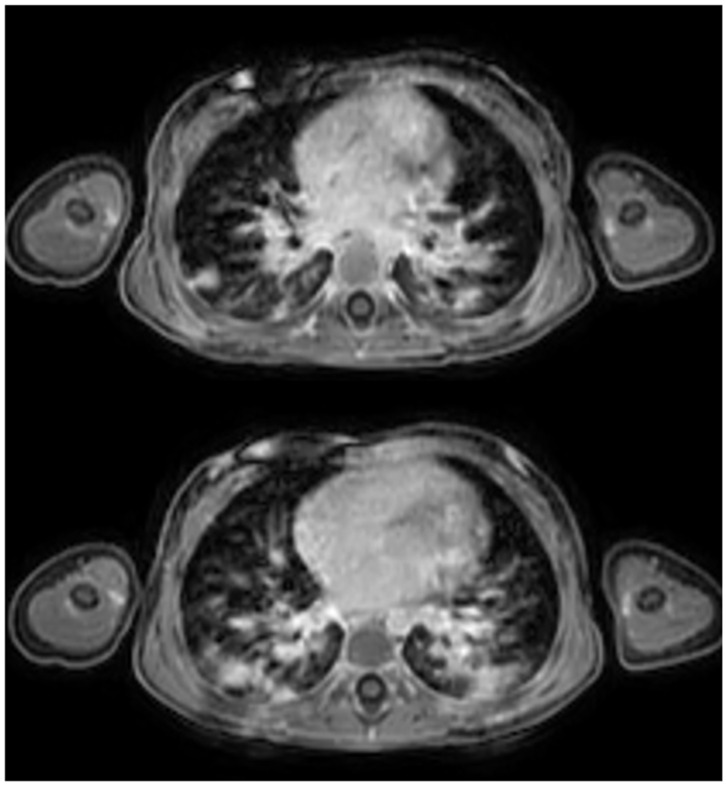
Post-contrast gradient-echo T1-weighted sequence of the chest in a 5-year-old boy, showing pneumonic consolidations in both lower lobes.
